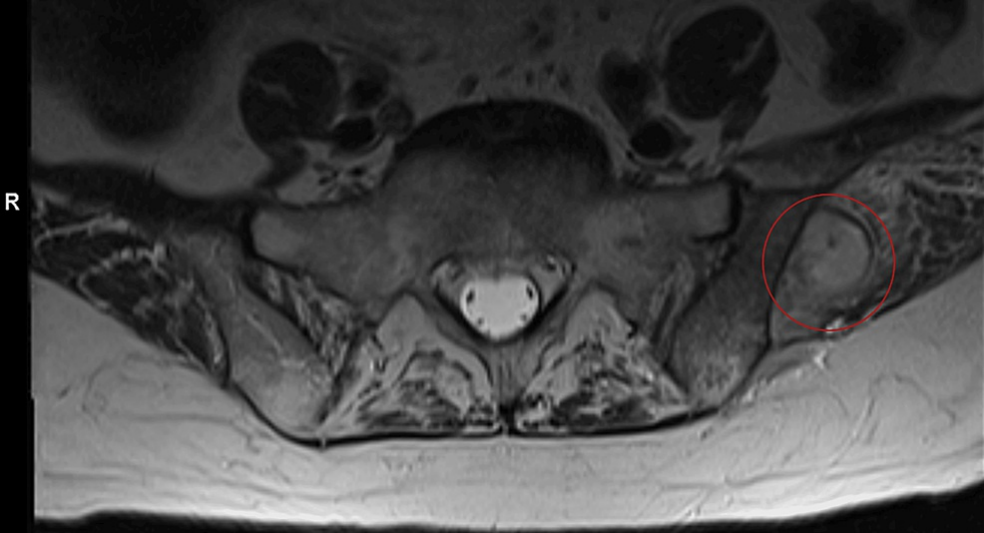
Lumbar MRI demonstrating osteomyelitis Potential abscess denoted with a red circle. The radiology report described acute osteomyelitis of the left iliac bone with multiple hypodensities surrounded by enhancement which may involve the left posterior paraspinal musculature at the level of L5. Suspicion for infectious process, possible abscess with probable sacroiliac involvement, and a lobulated gluteal abscess.
